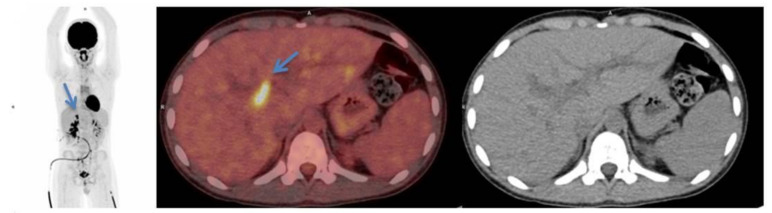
Initial scan: ^18^F-FDG-PET/CT maximal intensity projection (left), transaxial fused PET/CT image (middle) and CT image (right) of the ^18^F-FDG-avid lymph nodes in the liver hilum (blue arrows).
